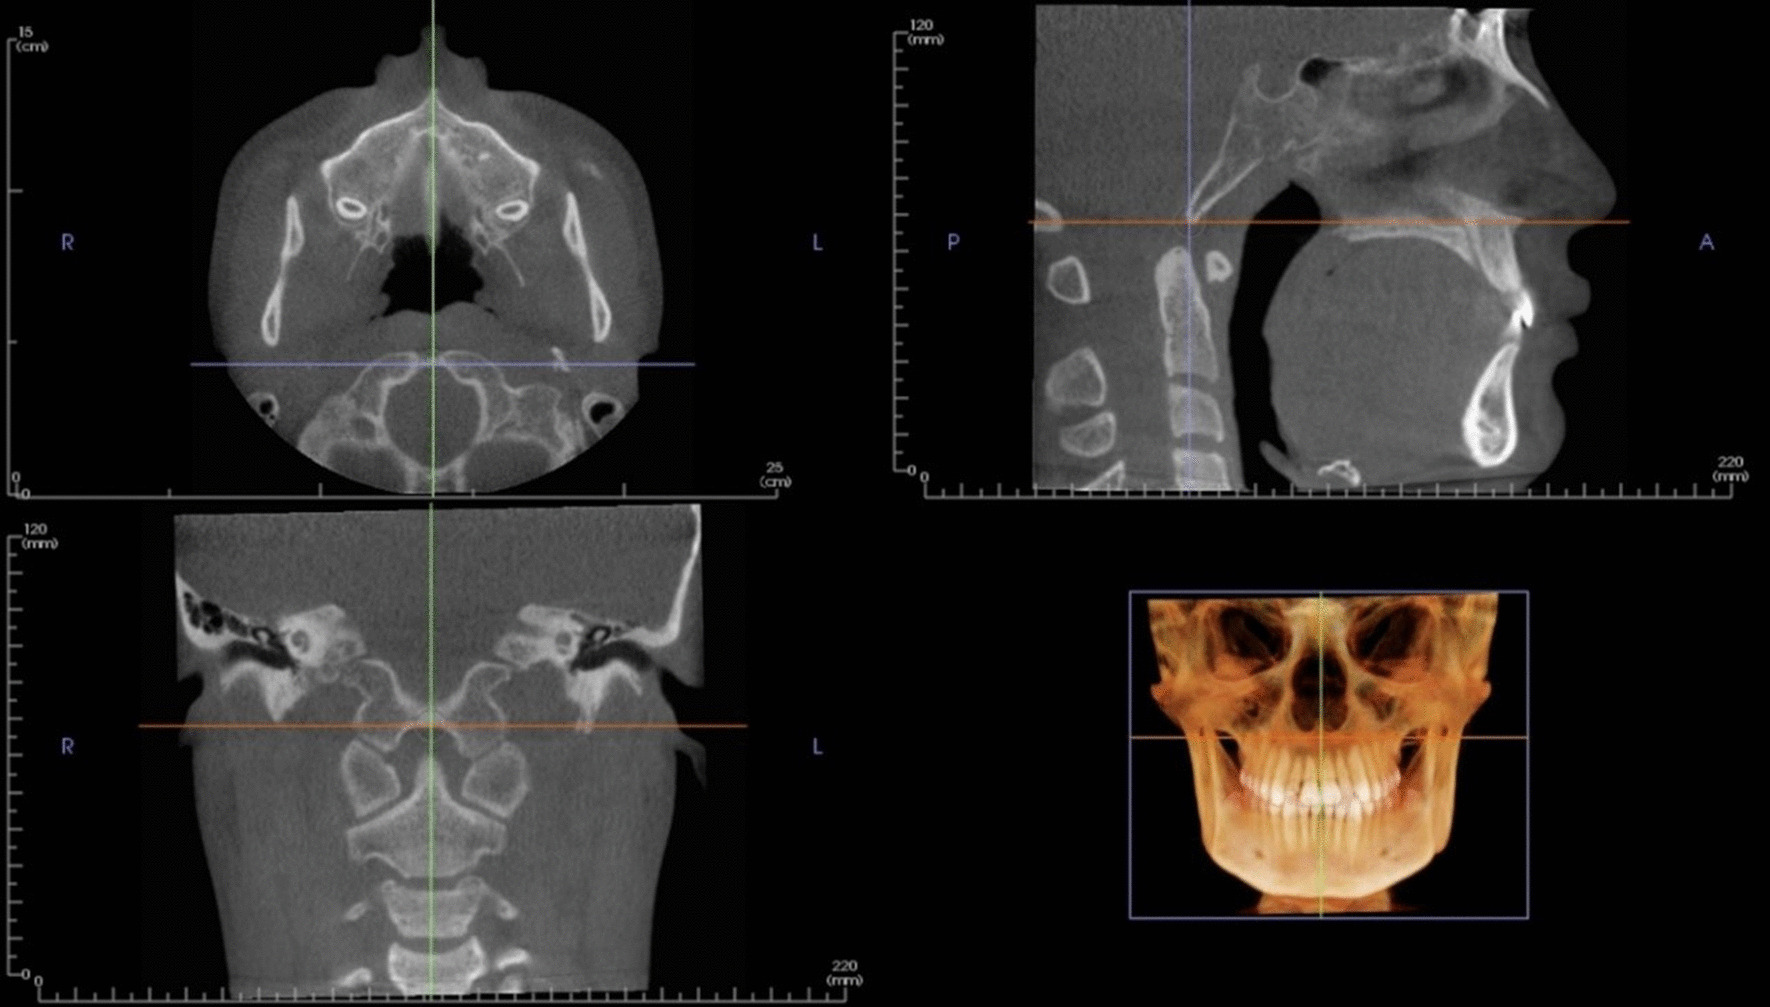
Mid-sagittal CBCT evaluation of spheno-occipital synchondrosis with the head in the default orientation
